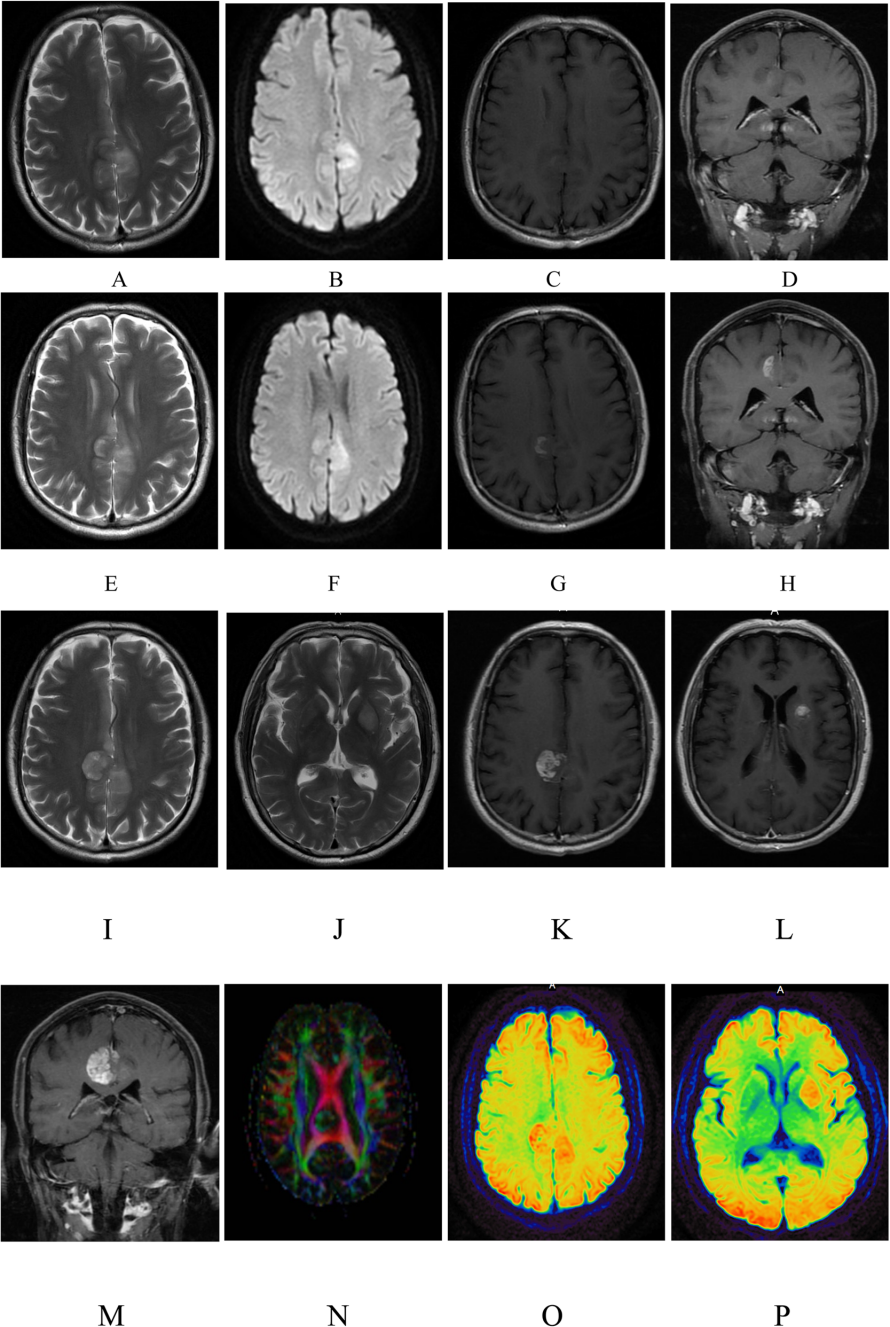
Brain MRI imaging of the patient. Brain MRI plain scan and enhanced scan (July 7, 2016) of the patient showed long T2 abnormal signal localized lesions (a) on the bilateral fronto parietal, proximal midline, Diffusion weighted imaging (DWI) high signal intensity (b), slight enhancement of lesions (c, d). Brain MRI plain scan and enhanced scan (September 18, 2016) of the patient showed bilateral fronto parietal, proximal midline, localized lesions long T2 signal(e), discrete wavelet transform (DWT) high signal high signal intensity (f), enhancement of lesions (g, h). Brain MRI plain scan and enhanced scan (November 15,2016) of the patient showed bilateral frontal and parietal lobes near the midline and left basal ganglia lesions long T2 signal(i, j), enhanced two lesions showed obvious enhancement (k, l, m), brain DTI showed partial fibrous bundle ablation of the bilateral frontal parietal lobe and left basal ganglia lesion. Fractional anisotropy (FA) decreased around the fiber bundle of mild compression and displacement(N). Arterial Spin Labeling (ASL): bilateral fronto parietal, left basal ganglia lesions were high perfusion (o, p)
